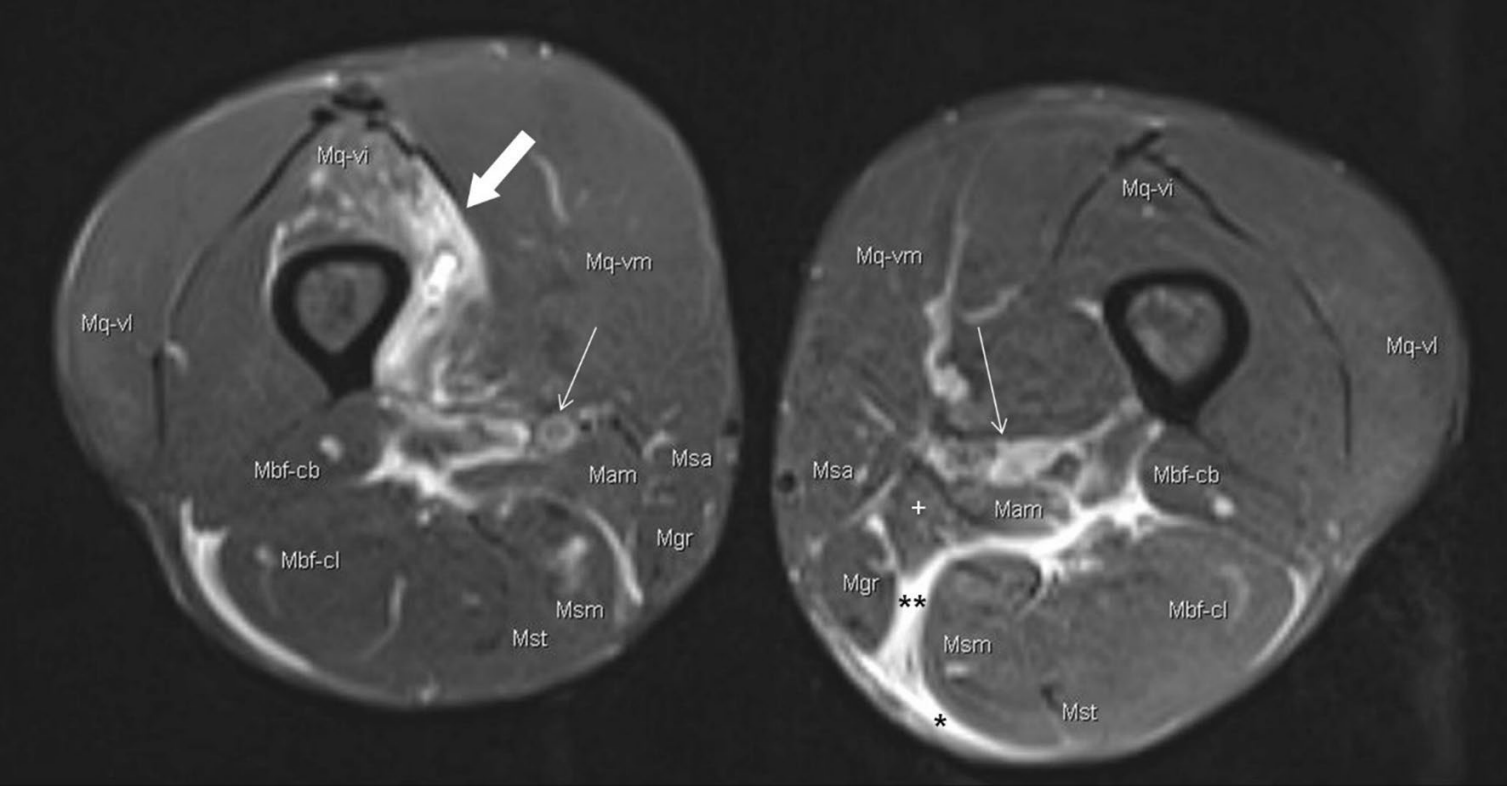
Water-sensitive MRI of upper legs (PDw: proton density weighting): muscle lesions and myofascial inflammation in the upper legs (56 years, male, stage 21 of TEFR, after 1521-km run). *Thick arrow*: muscle bundle rupture and myositis (M. quadriceps, Vastus intermedius); *thin arrow*: neurovascular bundle; * panniculitis, fasciitis;  intermuscular fasciitis. *Mq* M. quadriceps, *-vl* vastus lateralis, *-vi* vastus intermedius, *-vm* vastus medialis, *Mam* M. adductor magnus, *Msa* M. sartorius, *Mgr* M. gracilis, *Msm* M. semimembranosus, *Mst* M. semitendinosus, *Mbf* M. biceps femoris, *-cl* caput longum, *-cb* caput brevis
